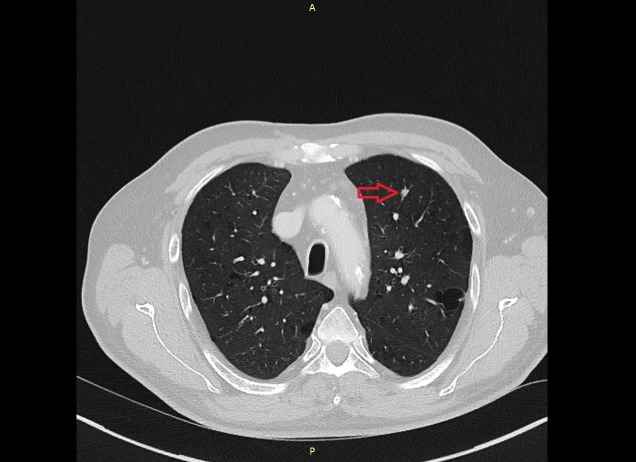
CT scan. The image shows the 6 x 6 mm lesion in the left upper lobe. CT: computed tomography
